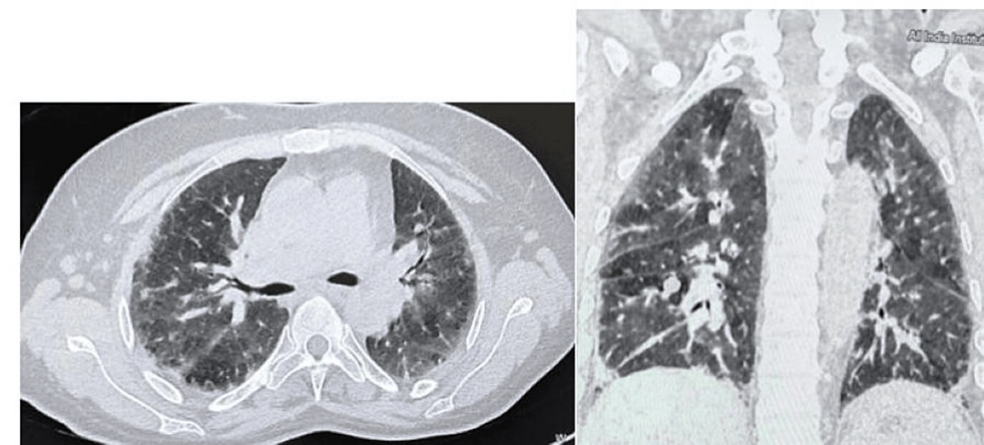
High-resolution CT scan of chest showing bilateral subpleural reticulations with ground glass opacities with sub-centimetric mediastinal lymph nodes with calcified lung nodules with surrounding cysts in bilateral upper lobes and right lower lobe suggestive of interstitial lung disease.
